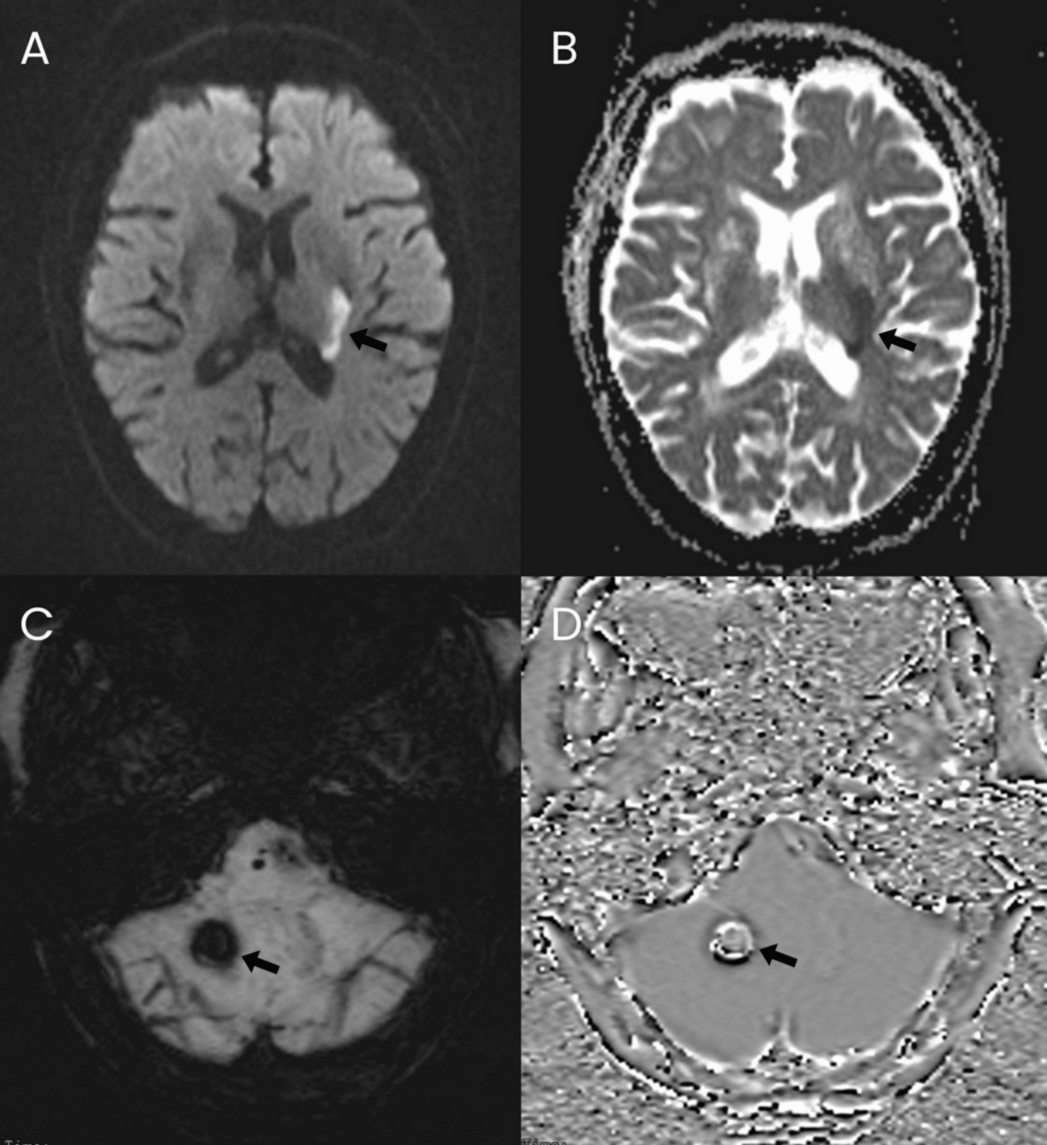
MRI findings. Axial DWI (A) and ADC (B) show restricted diffusion involving the left caudate body, posterior limb of the internal capsule, and lentiform nucleus (arrows), consistent with acute infarction. SWI (C) and filtered-phase imaging (D) demonstrate a small acute hemorrhage in the inferior right cerebellar hemisphere (arrows). Abbreviations: DWI, diffusion-weighted imaging; ADC, apparent diffusion coefficient; SWI, susceptibility-weighted imaging.
